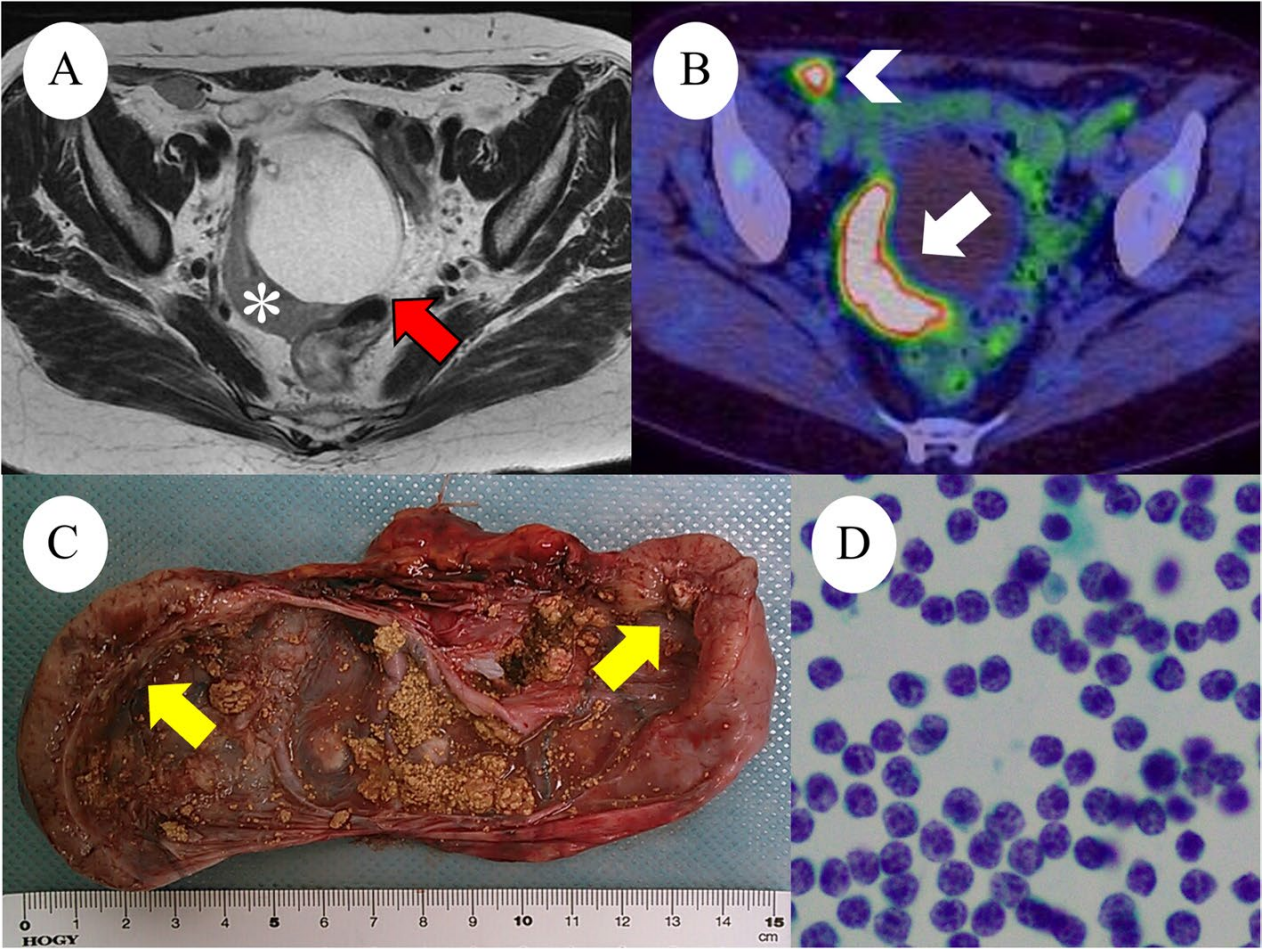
A: Axial T2-weighted magnetic resonance image reveals a 70-mm right ovarian mass (red arrows) with a 20-mm crescent solid part at the dorsal side (white asterisks). B: Positron emission tomography-CT shows FDG accumulation in the solid component (white arrows), measuring SUV max od 8.3 and retroperitoneal lymph node (white arrowhead), measuring SUV max of 7.8. C: Right ovarian tumor, macroscopic examination shows cystic tumor filled with fatty and yellow sebaceous component and solid nodule (yellow arrows). D: Ascites cytology was no neoplastic findings
